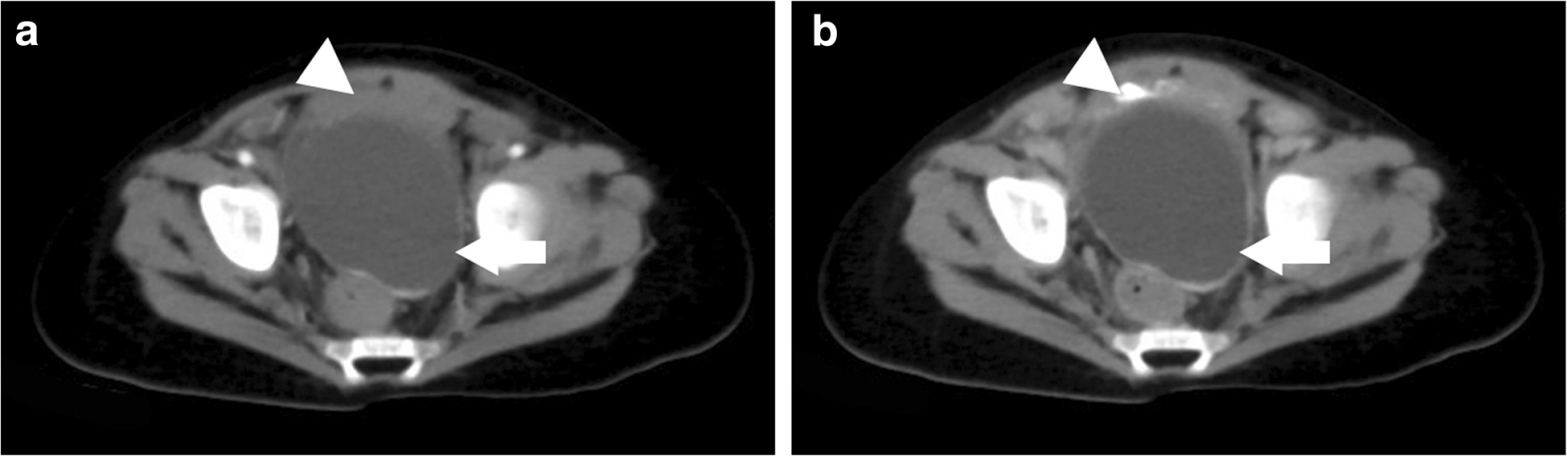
Contrast-enhanced computed tomography shows a large, utricular mass (white arrow) behind the urinary bladder (white arrowhead). a Enhanced CT shows a utricular retrovesical mass compressing the urinary bladder. b Delayed enhanced CT shows the bladder filled with contrast agent, a large utricular mass located behind and in close proximity to the urinary bladder
